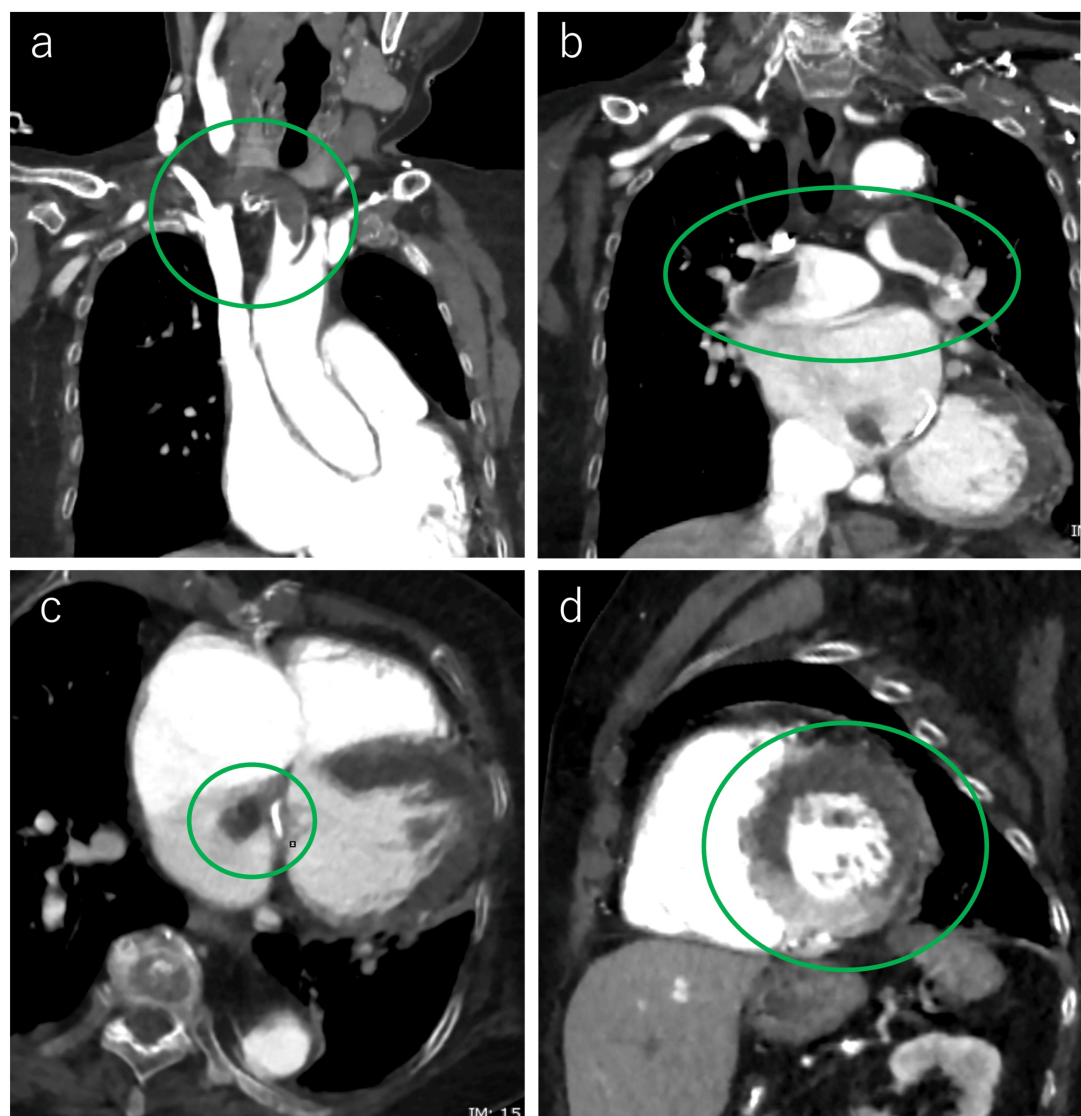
Whole-body CT findings with the lesion indicated by green circles. (a) Coronal image showing thromboembolism at the origin of the brachiocephalic artery, considered the cause of cerebral infarction. (b) Coronal image showing massive thrombi in the main trunks of the left and right pulmonary arteries, indicating pulmonary thromboembolism. (c) Axial image showing a thrombus in the left atrium near the mitral valve. (d) Short-axis cardiac image showing reduced contrast enhancement of the myocardium wall, raising suspicion of myocardial infarction.
